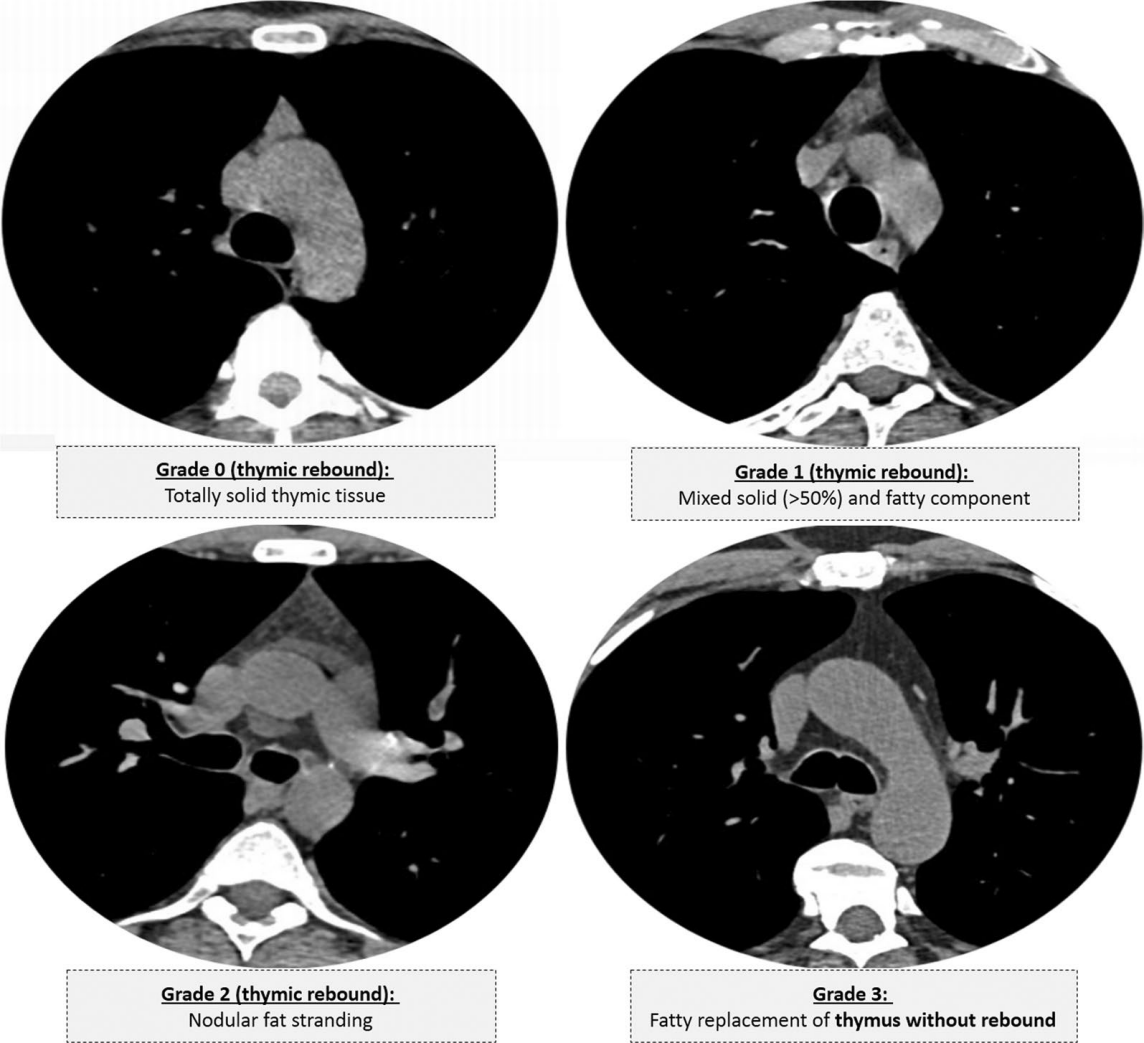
Thymic CT-grading (according to Cuvelier et al. [5]) with provided examples for different CT images with thymic CT-grades (0 to 3) from the current study
